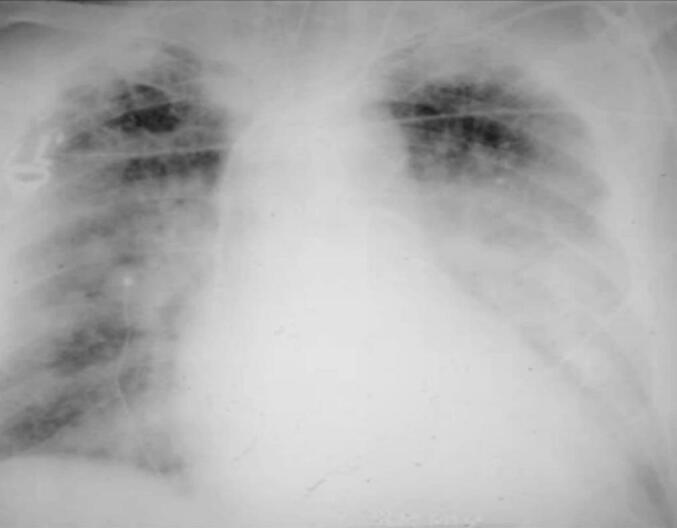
A chest X-ray showing an enormous cardiomegaly.
